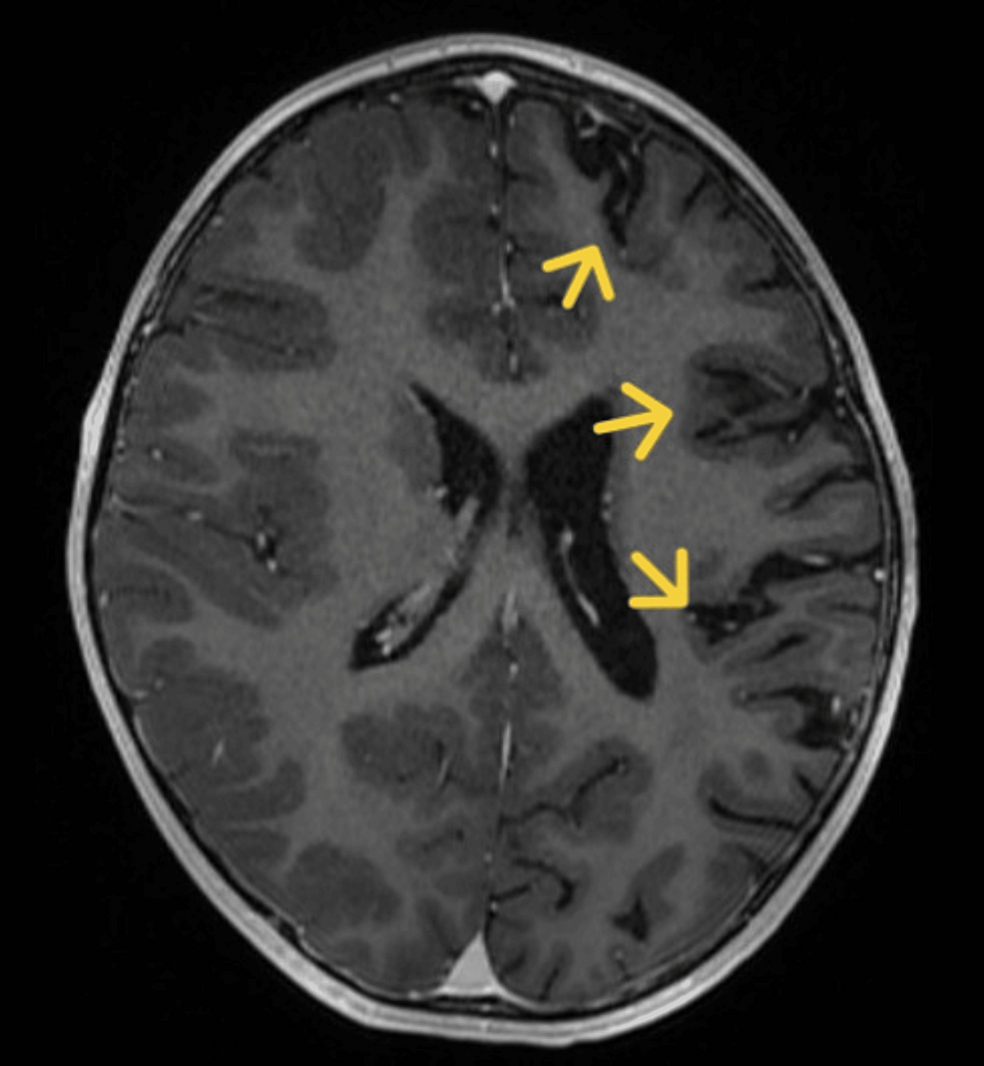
Second brain MRI with contrast Decrease of the left hemisphere volume. Note that the frontal, parietal, and temporal lobes are the most affected as observed in the first MRI.
